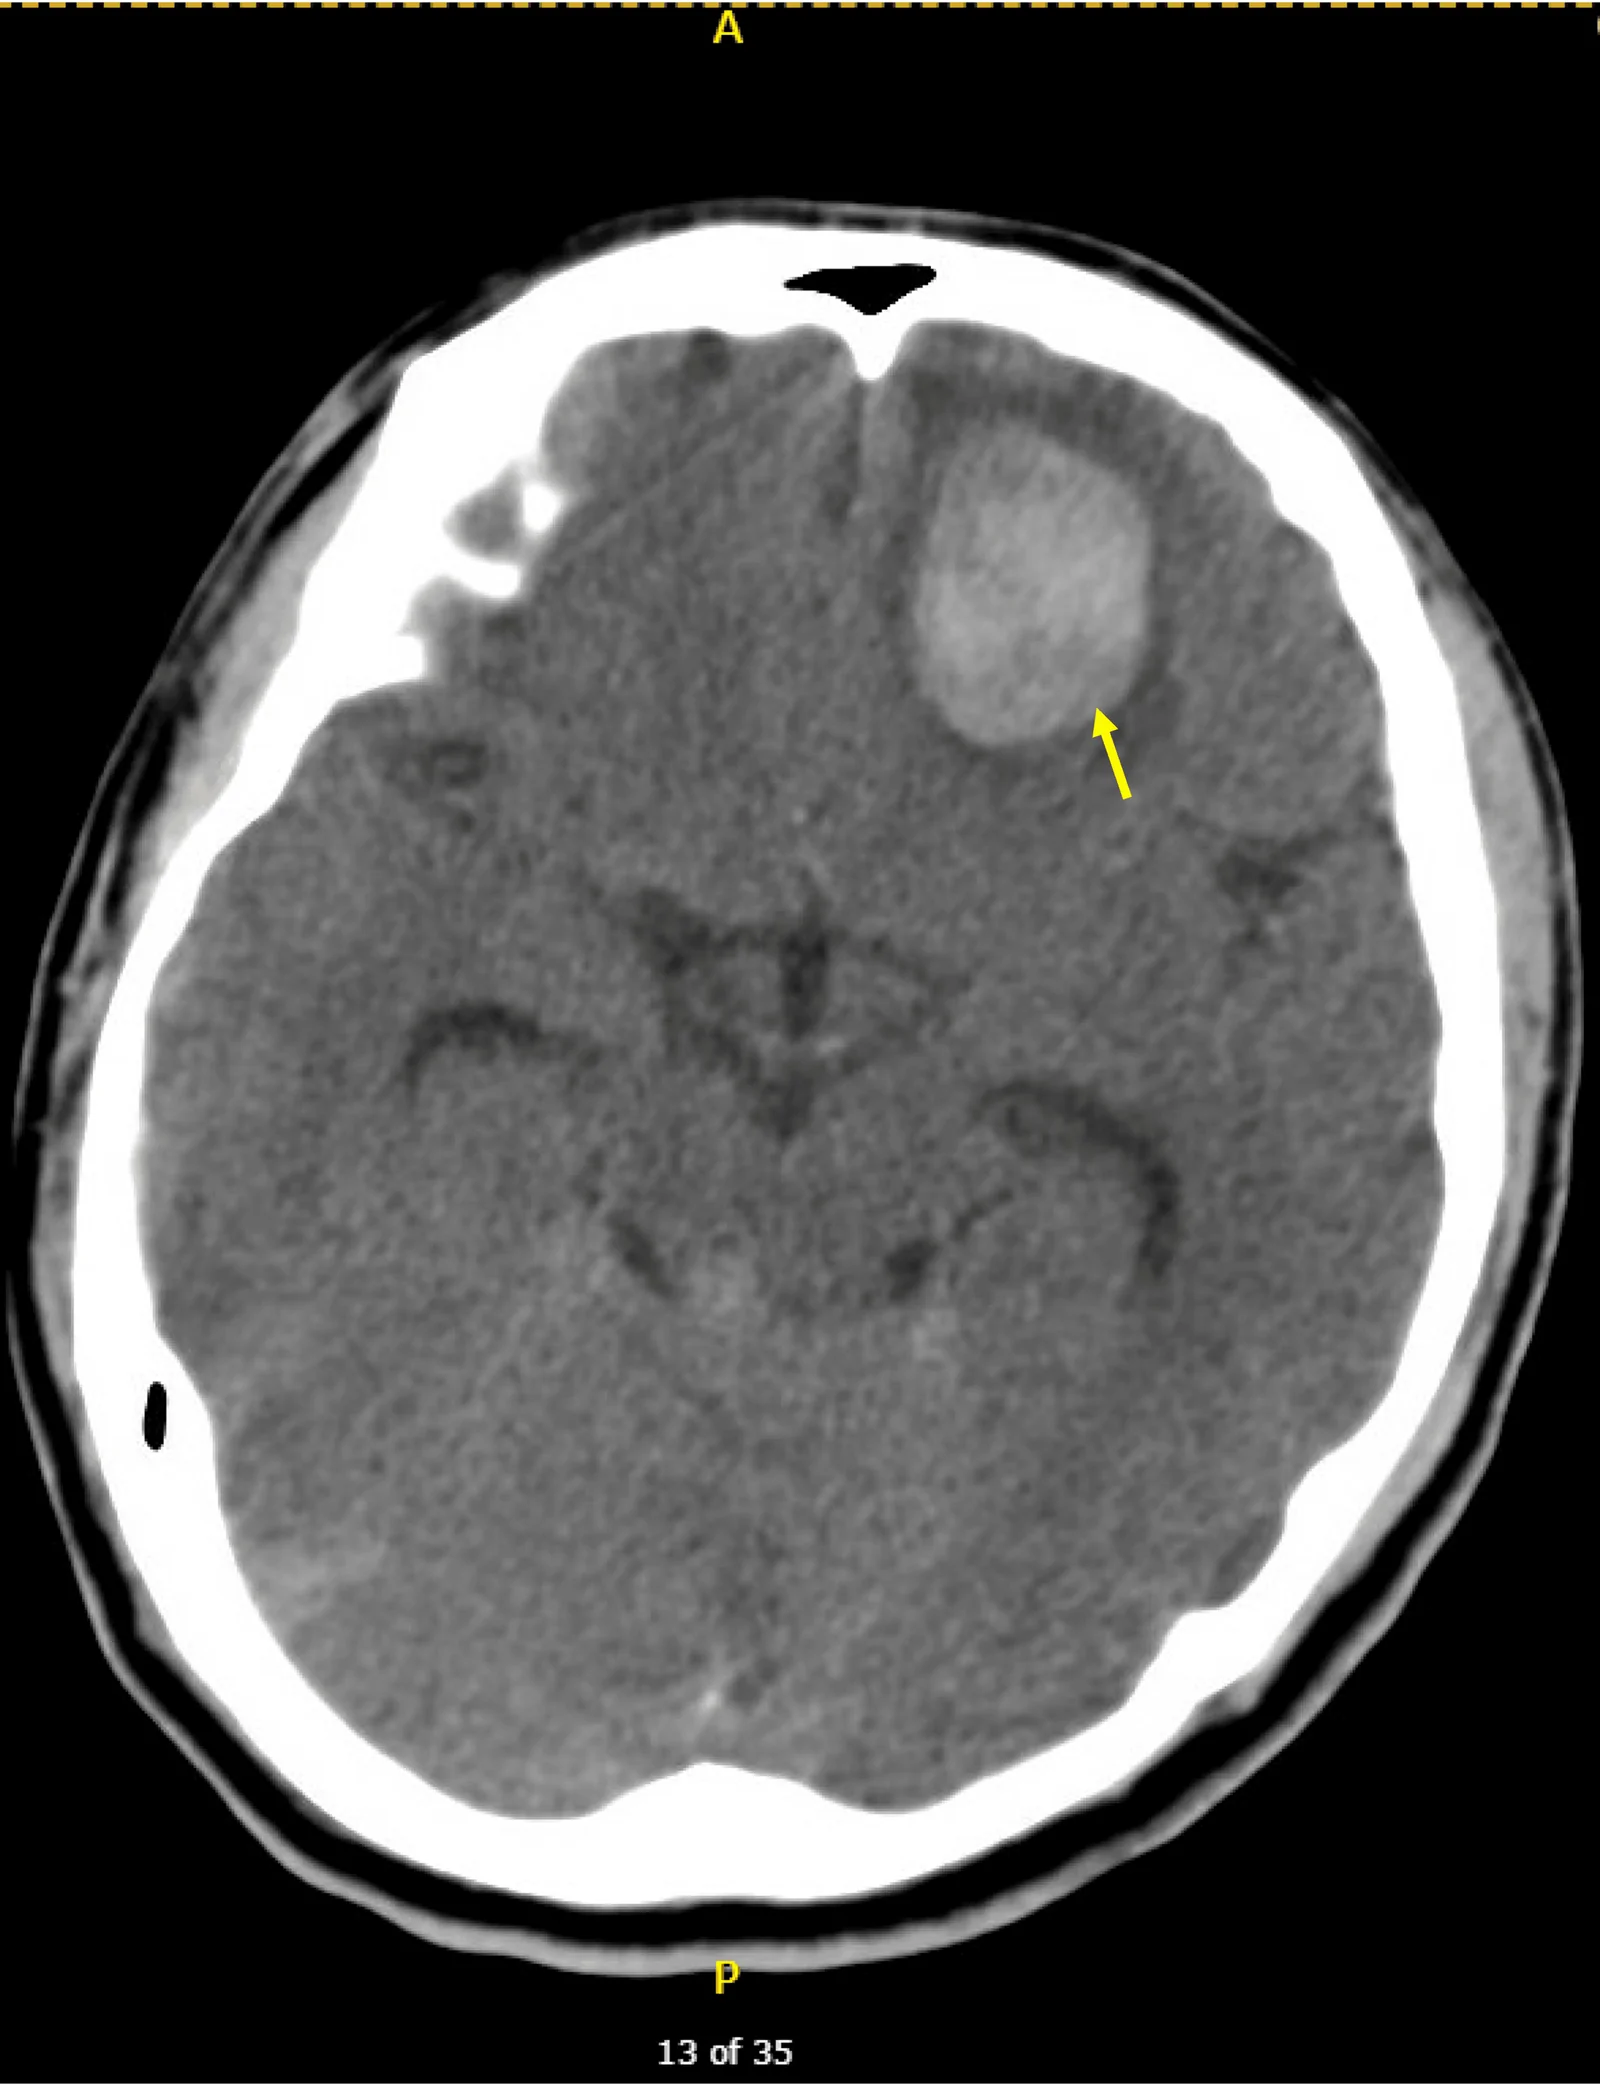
Non-contrast CT of the brain demonstrates new hemorrhage Representative axial slice from the second admission, four days after discharge from the first. Arrow points to a new parenchymal hemorrhage in the superior left frontal lobe; additional new hemorrhages (inferior left frontal lobe, right cerebellum) and new SAH (right parietal convexity) were also identified on this study but are not shown. This recurrence despite a normal aPTT prompted reassessment of the presumed VWD diagnosis and ultimately led to the diagnosis of severe FXIII deficiency. SAH: subarachnoid hemorrhage; aPTT: activated partial thromboplastin time; FXIII: Factor XIII; CT: computed tomography; VWD: von Willebrand disease
